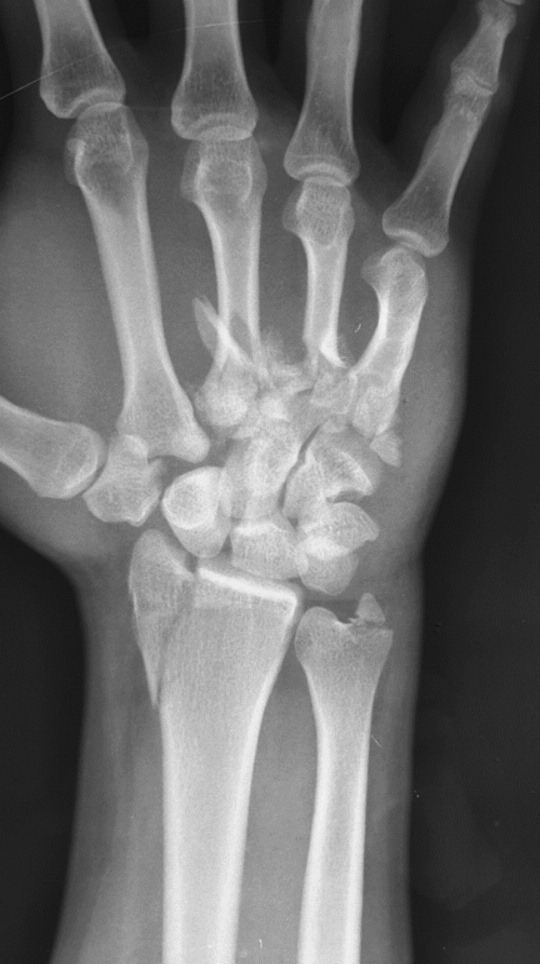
Radiograph shows the injuries of patient’s right hand.
